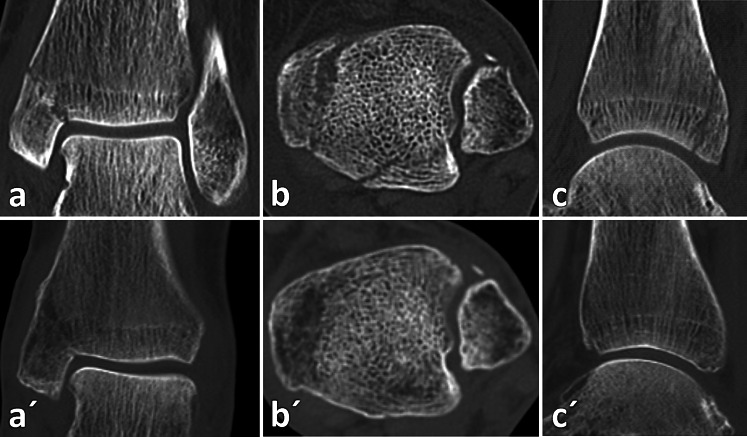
Patient N-12. a; b; c – frontal, axial and sagittal postinjury CT scans demonstrate a nondisplaced bicollicular fracture of MM, a nondisplaced fracture of PM type 2 according to B-R classification and 10 degree external rotation of the distal fibula in FN; a; b; c – frontal, axial and sagittal CT scans 40 months after injury, all fractures healed in an anatomical position, external rotation of distal fibula improved
